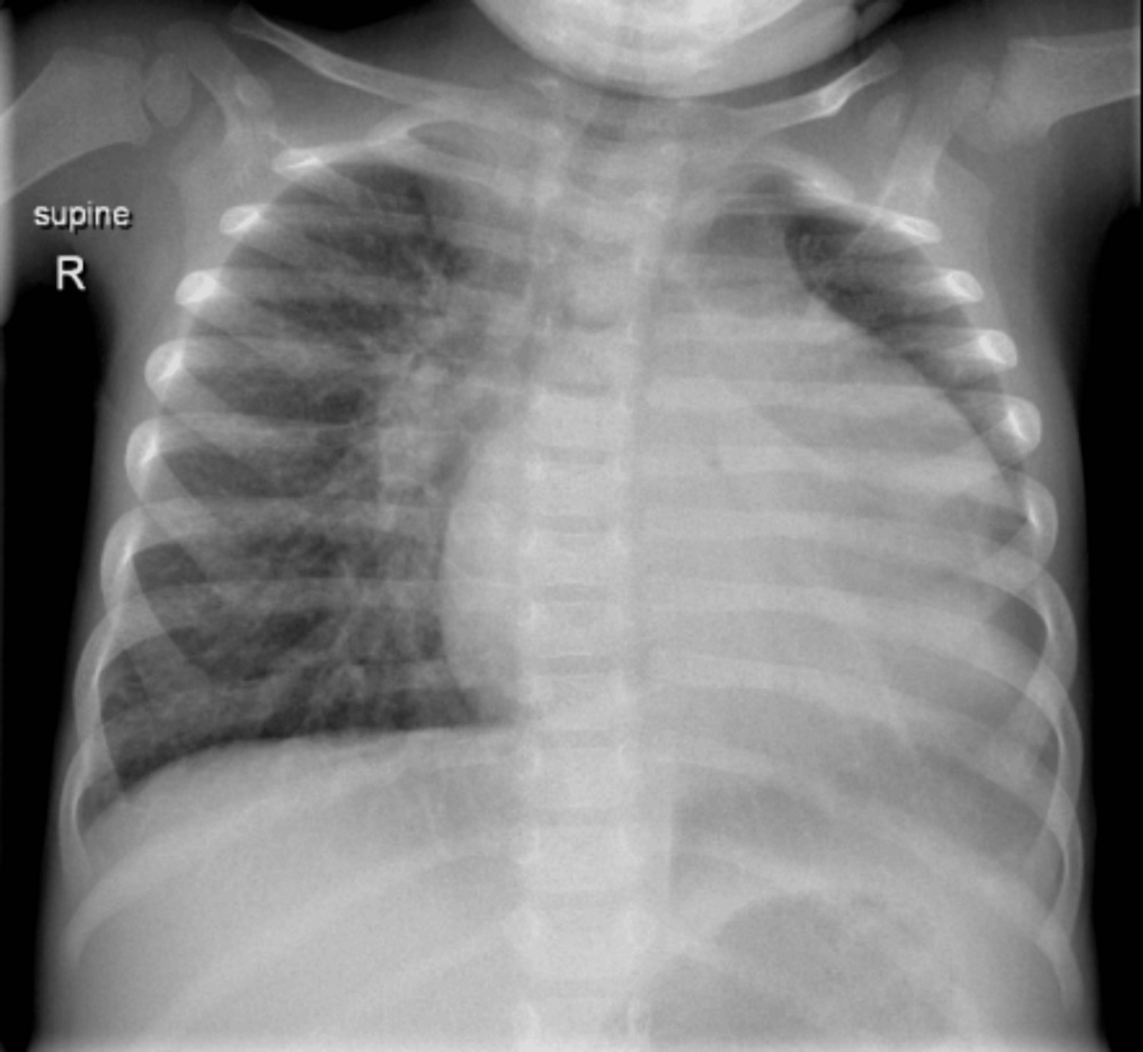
At presentation the chest X-ray shows cardiomegaly
